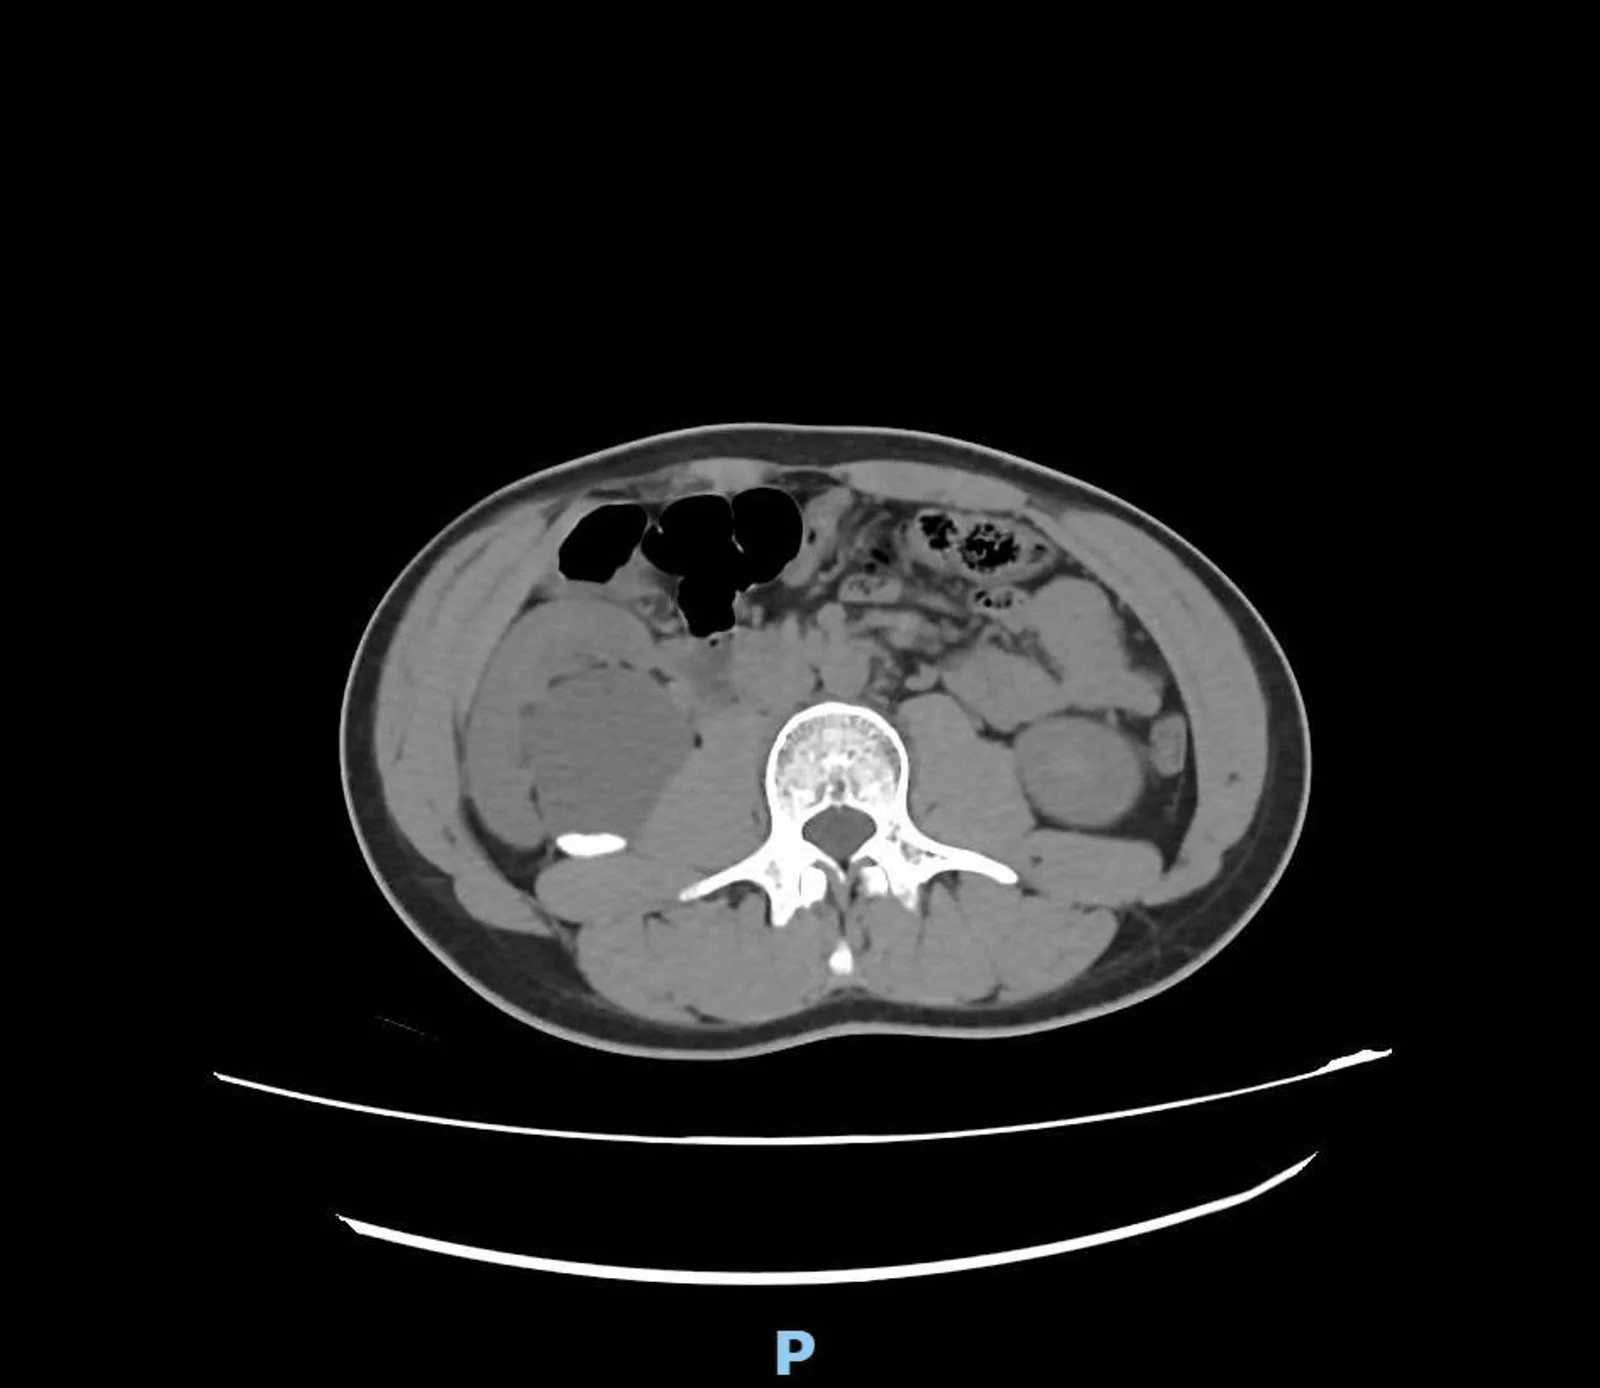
Right Renal Pelvicalyceal Dilatation With Renal-Pelvic Calculus. Contrast-enhanced axial CT image demonstrating marked right pelvicalyceal dilatation and a hyperdense renal-pelvic calculus. Associated cortical thinning and absence of ureteric dilatation contributed to the radiologic impression of pelviureteric junction stenosis.
